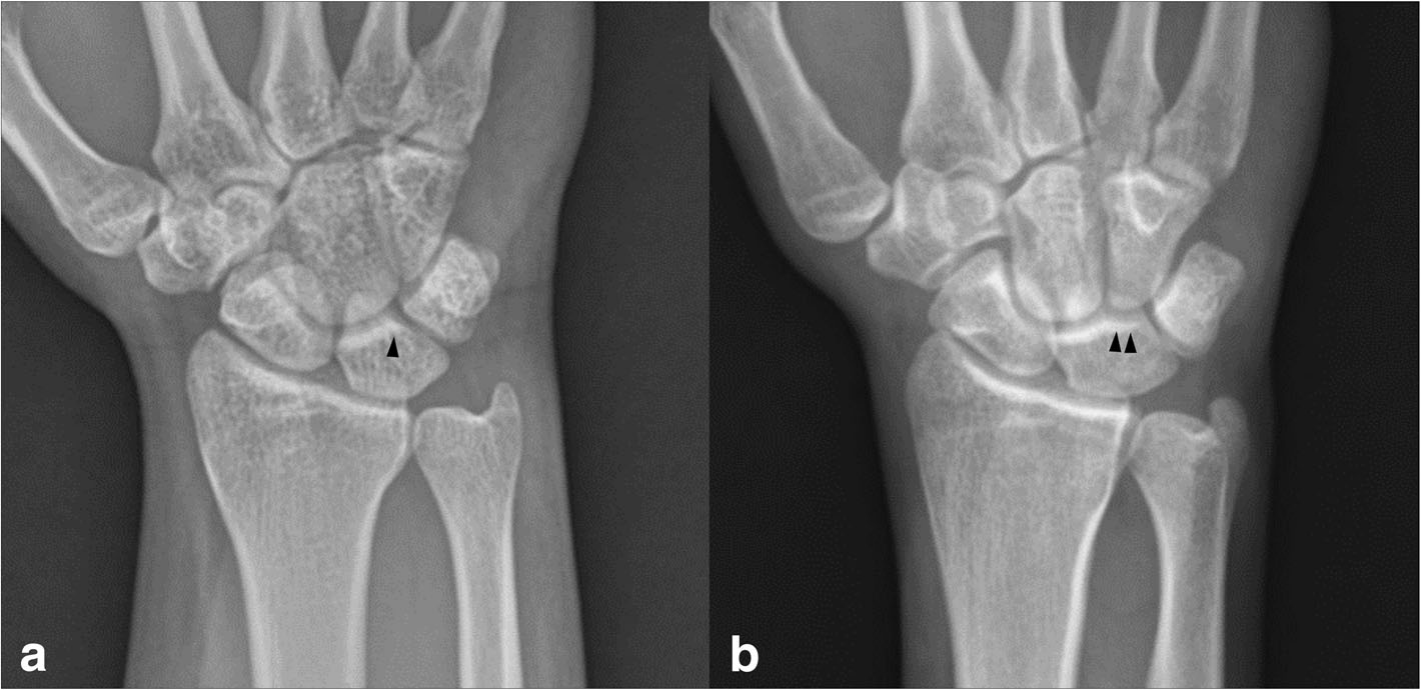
Examples of (a) Type I and (b) Type II lunates classified by plain radiographic analysis; arrowheads point to the absence or presence of a medial hamate facet on the lunate, respectively
